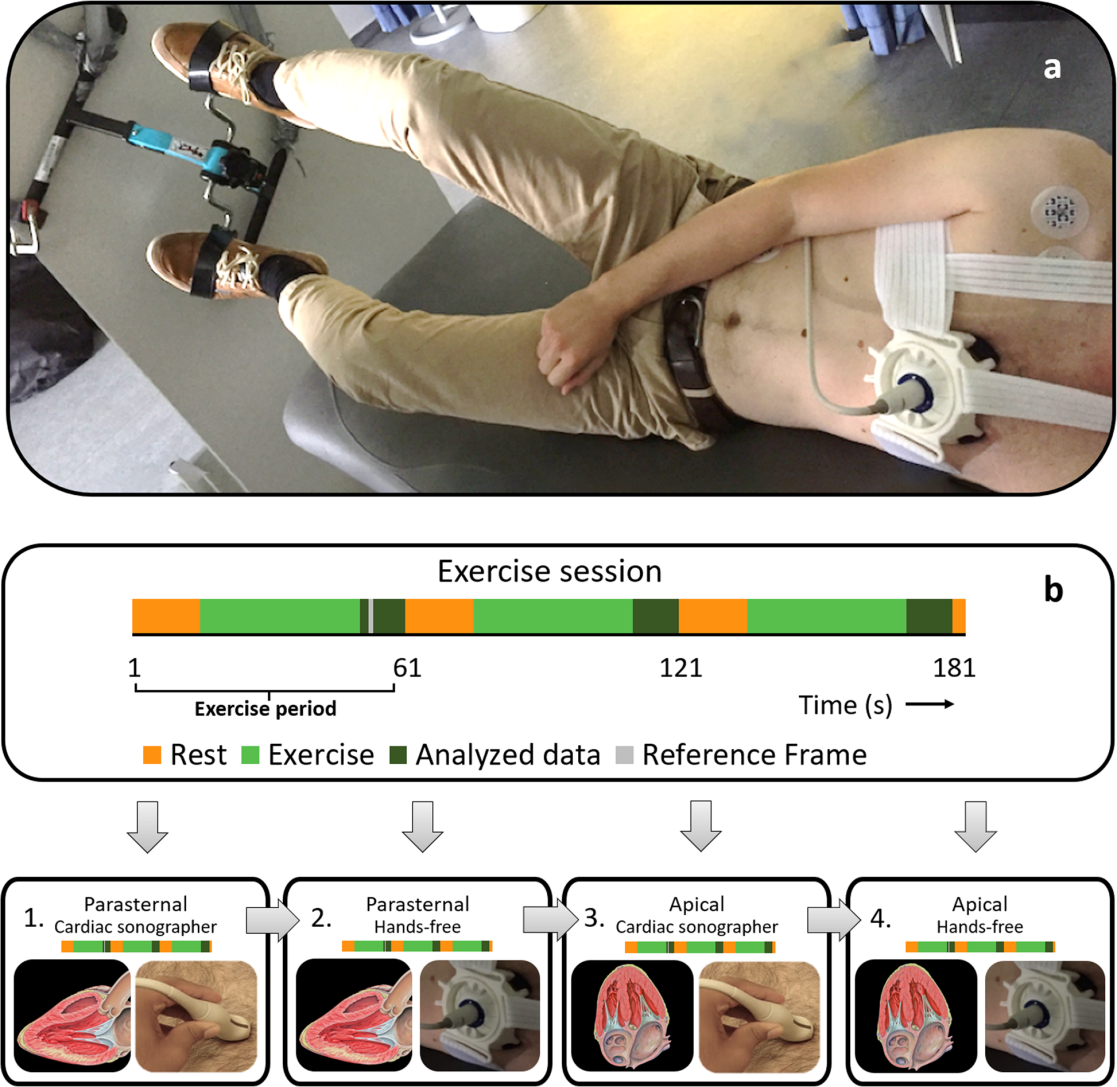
Experimental setup and acquisition protocol. a The semi-supine exercise setup in which the probe is fixated to acquire hands-free images of the heart. b The acquisition protocol. (1) Images were acquired manually in the parasternal view, followed by (2) hands-free measurements. Subsequently, (3) the manual and (4) hands-free acquisitions were obtained in the apical view [26–28]. The images of the last ten seconds of every exercise period were used for further assessment
